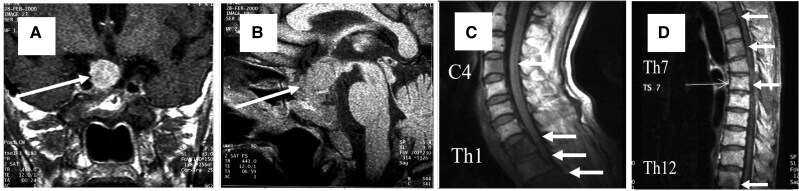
Pituitary and spinal magnetic resonance imaging (MRI) findings. A, Coronal pituitary MRI at onset. B, Sagittal pituitary MRI at onset. C, Cervical spine MRI at 7 years from onset, showing multiple bone metastases. D, Thoracic spine MRI at 7 years from onset, also demonstrating multiple bone metastases (C4, Th1–3, Th5, Th7, Th12, L2–5, and S1). Abbreviations: C, cervical; L, lumbar; S, sacral; Th, thoracic.
